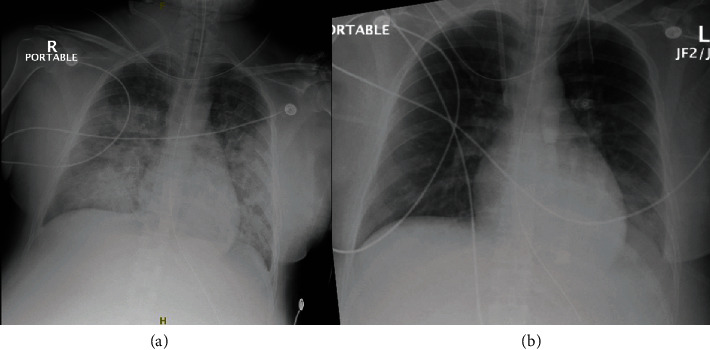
(a) Chest X-ray immediately postoperation demonstrating bilateral diffuse airway opacities demonstrative of acute respiratory distress syndrome. (b) Chest X-ray 72 hours following the index X-ray demonstrating complete resolution of bilateral airway opacities.
